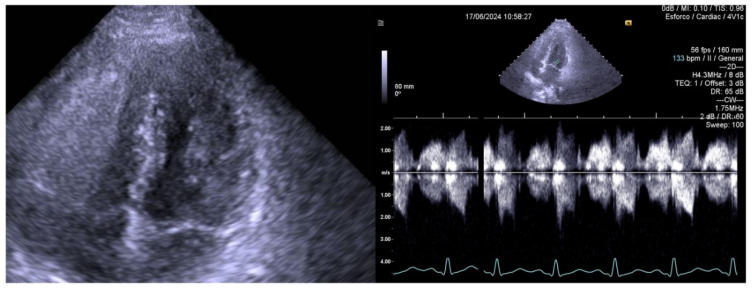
ECHO at the end of exercise stress echocardiography on bisoprolol 2.5 mg without SAM, without intraventricular gradient, and also without angina.
